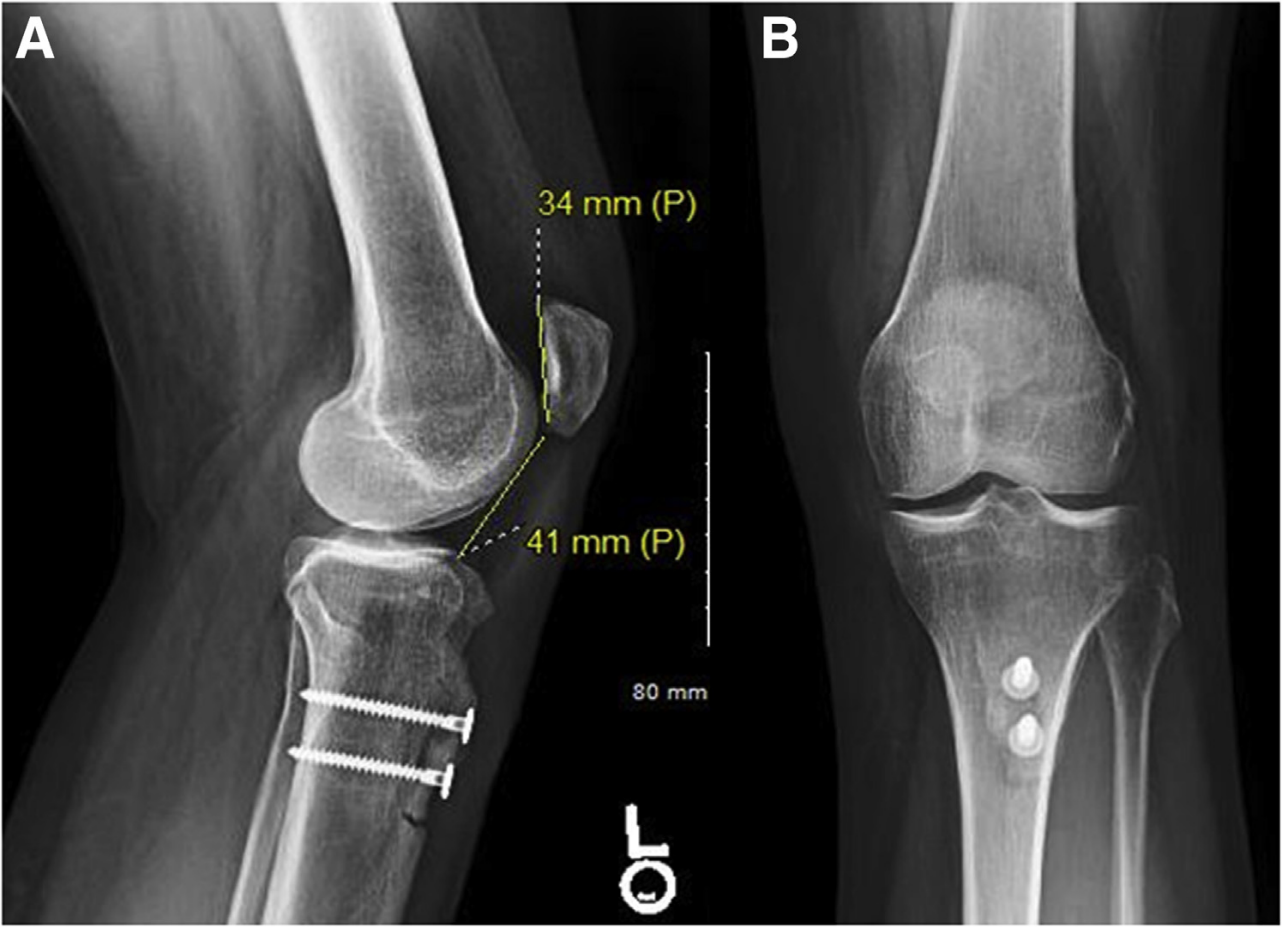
Postoperative radiographs of a left knee after tibial tubercle distalization osteotomy. (A) Lateral radiograph showing improved patellar height with Caton-Deschamps index of 1.2. (B) Anteroposterior radiograph showing final screw location and osteotomy position.
